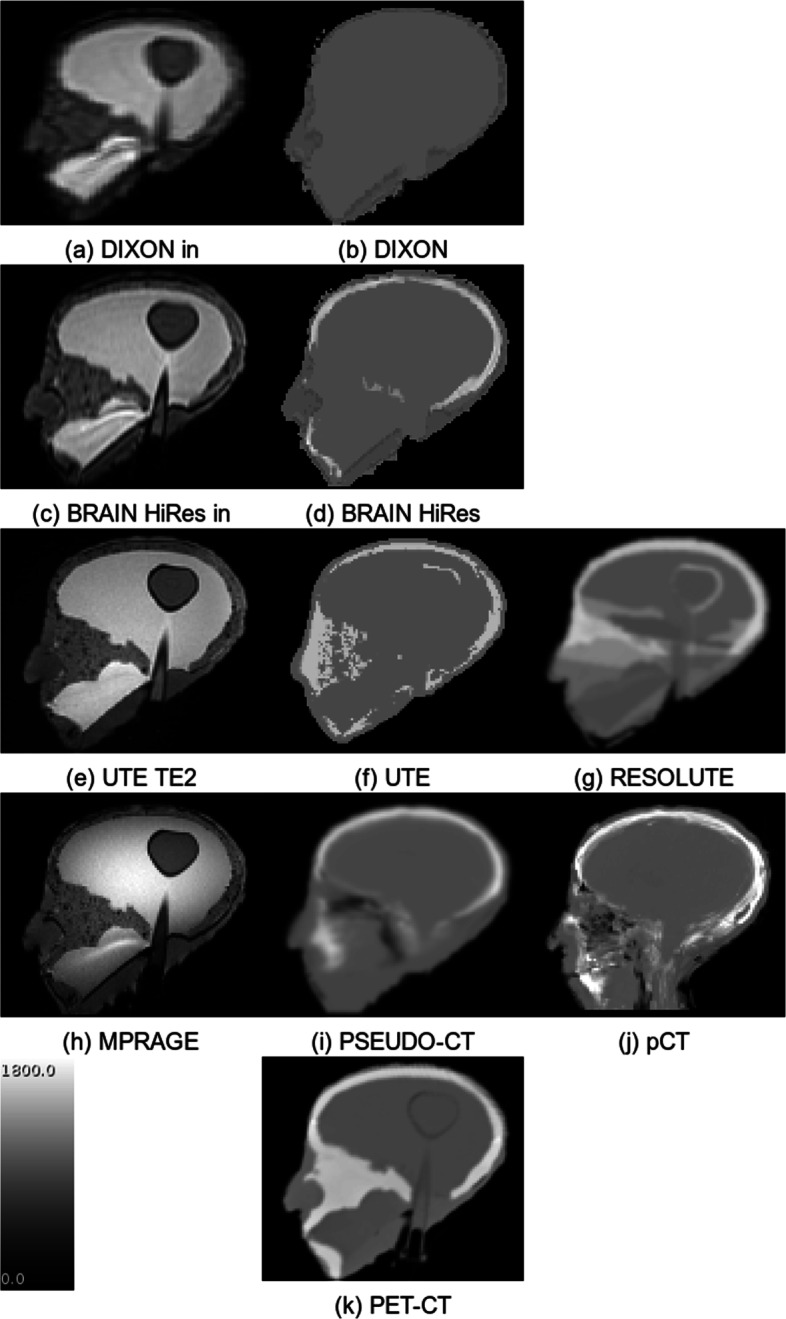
MRI data (leftmost column) and *μ*-maps derived from it (other columns). a, c The magnitude of the in-phase Dixon data and e the second echo of the UTE sequence. The *μ*-maps are labeled the same as in Table 1. For comparison, k displays the *μ*-map derived from the CT data of the PET-CT examination
